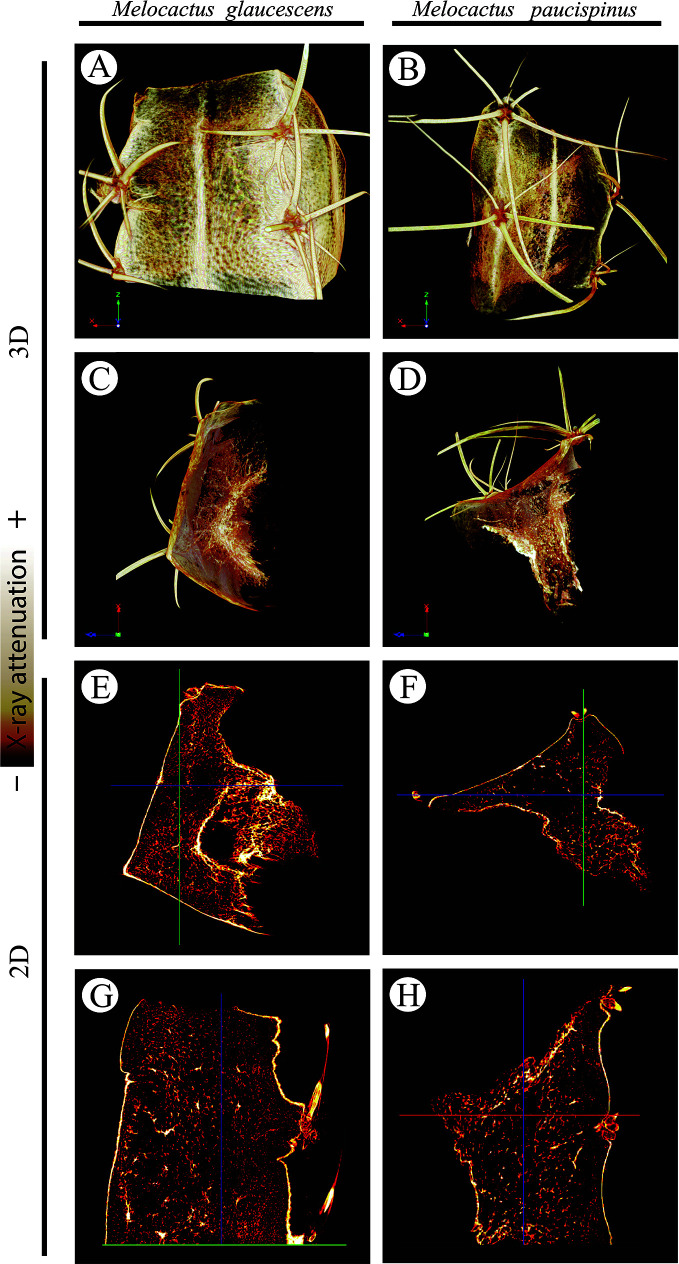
Three- (3D; A–D) two-dimensional (2D; E–H) reconstructions of *Melocactus glaucescens* (left column) and *Melocactus paucispinus* (right column) stems by X-ray micro-computed tomography, relying on the differential X-ray attenuation by the lumen and cell walls.
